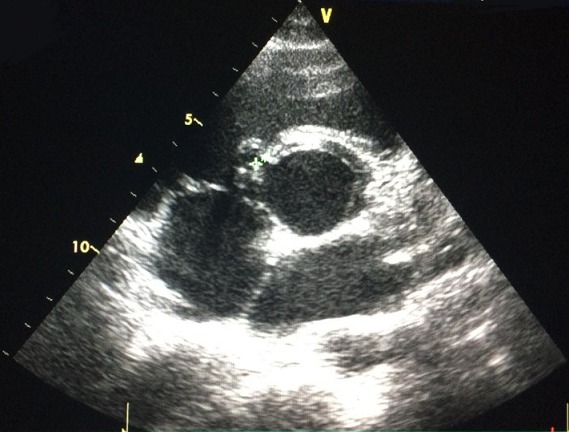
Incidence parasternale petit axe montrant une CIV périmembraneuse de 3 mm
